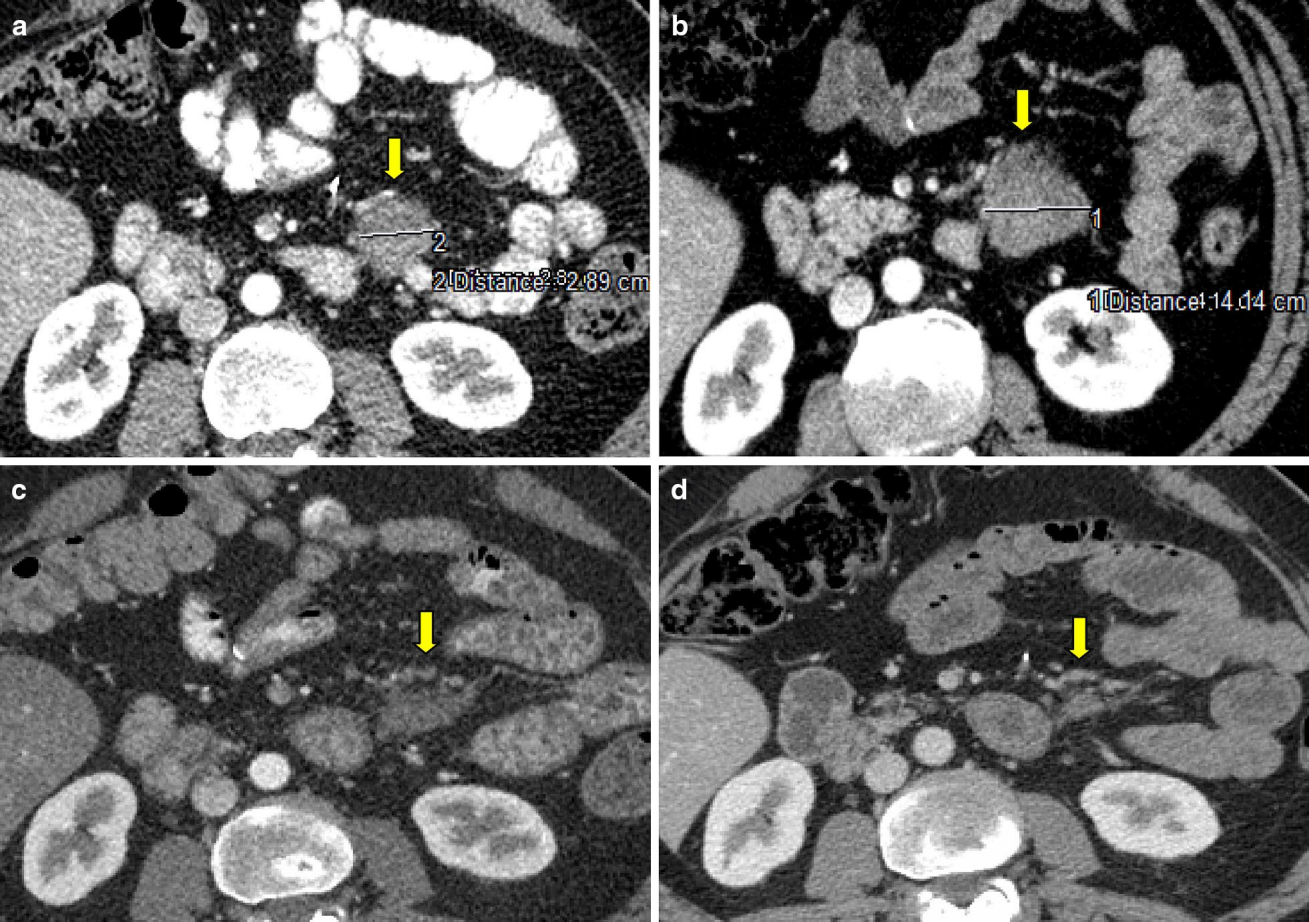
Patient CT scans; a before initial treatment of tamoxifen; axial plan CT scan performed in September 2013 demonstrates 2.89 cm diameter homogeneous mass located at the *left* anterior pararenal space of the retroperitoneal area (*yellow arrow*). b Post contrast CT images in december 2013; retroperitoneal mass (diameter 4.14 cm) progressed after treatment of tamoxifen (*yellow arrow*). c 9 months after the initiation of pazopanib treatment; the residual mass regressed on CT scan at same level (*yellow arrow*). d Post contrast CT images obtained in October 2015 showing complete response of the residual mass at 22 months of pazopanib treatment.(*yellow arrow*)
